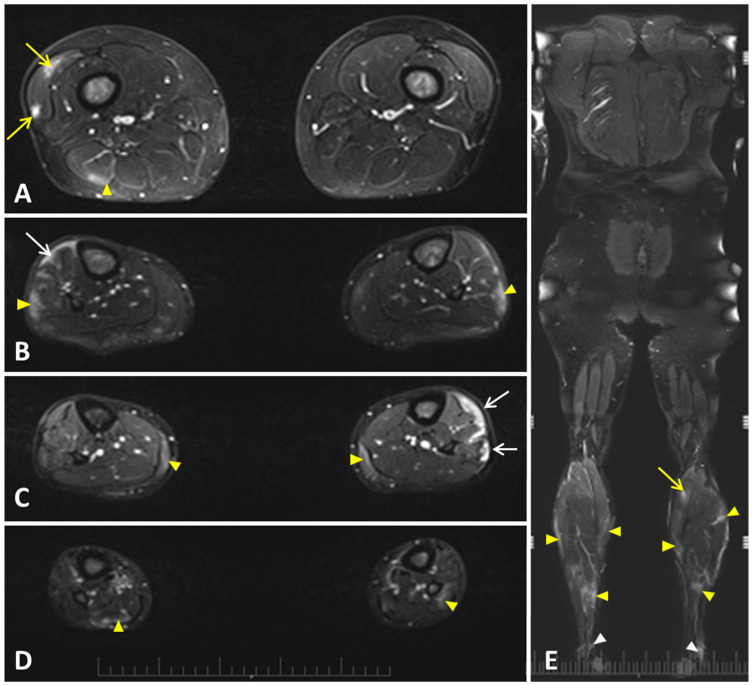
Cranio-caudal axial STIR-weighted whole body magnetic resonance imaging (WBMRI) of the thighs (A) and legs (B–D), and a coronal section of the entire body (E) of our patient with familial Mediterranean fever and protracted febrile myalgia. Three different patterns of hyperintense areas suggesting edema were detected: (1) Tubular-rounded foci within the muscle of the thigh (long yellow arrows) involving the right vastus lateralis (A) and the left gastrocnemius (E), with hiperintensity of the medullary bone (A); (2) Subfascial pseudocollections (long white arrows) involving right deep fascia-tibialis anterior and extensor digitorum longus muscles (B) and the same level of the opposite leg, including the leg fibularis muscle (C); and (3) Subtle, cotton-like miofascial foci (yellow arrowheads) affecting the right biceps femoral muscle (A), bilateral fibularis muscles (B,E), bilateral medial head of gastrocnemius (C,E), and right soleus muscle and left fibularis mucle (D,E). Hyperintense signal of both Achilles tendons (white arrowheads) is also observed (E).
